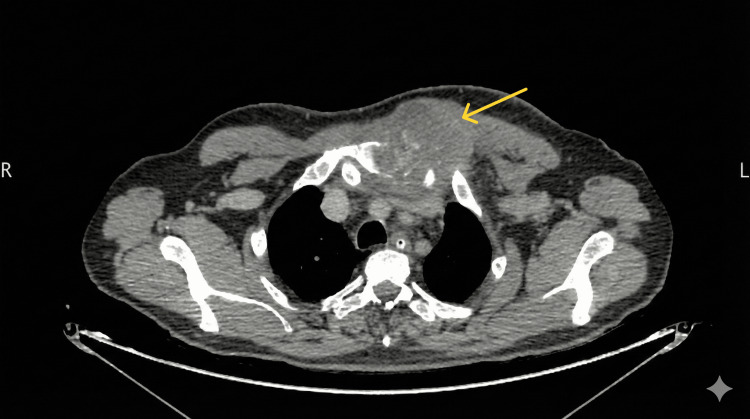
Axial CT scan image shows the sternal mass of Case 2. The yellow arrow depicts the sternal mass.
